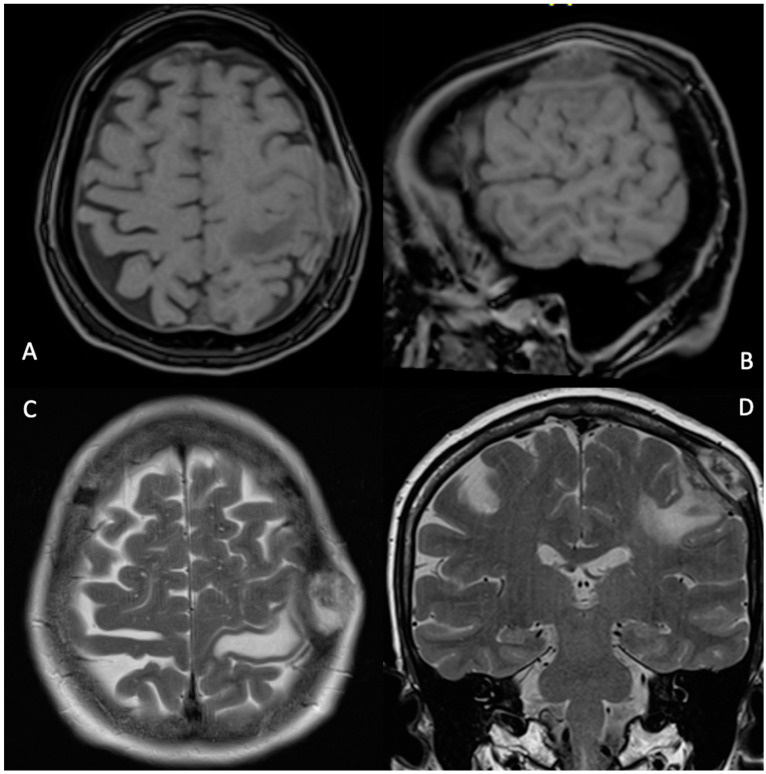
MRI (A) axial and (B) sagittal T1-weighted scans showing intra-calverian metastasis; (C) axial and (D) sagittal T2-weighted scans showing the lesion and the related edema in motor area.
